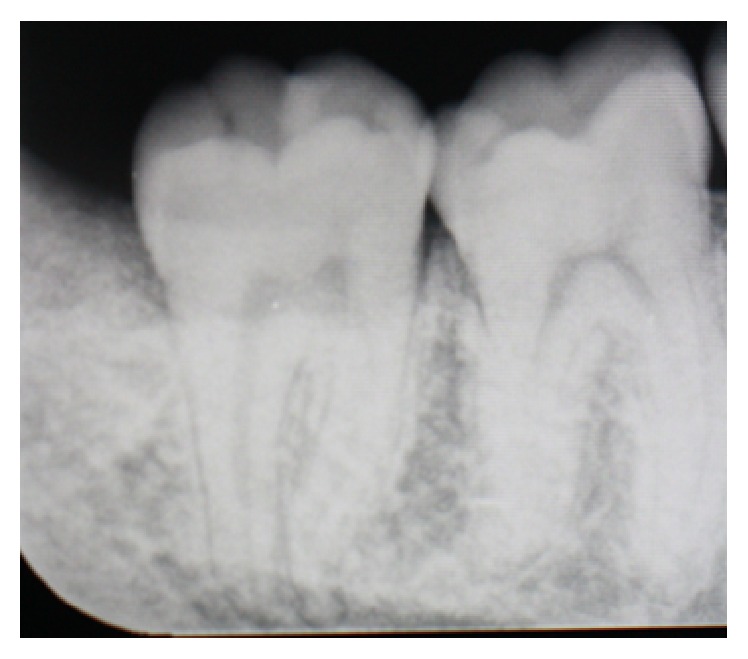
Postoperative periapical X-ray of the lower right second molar.
